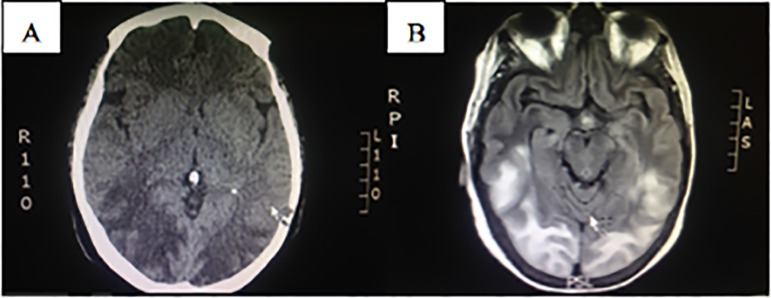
A. Brain CT scan disclosing extensive hypo-density at the sub
